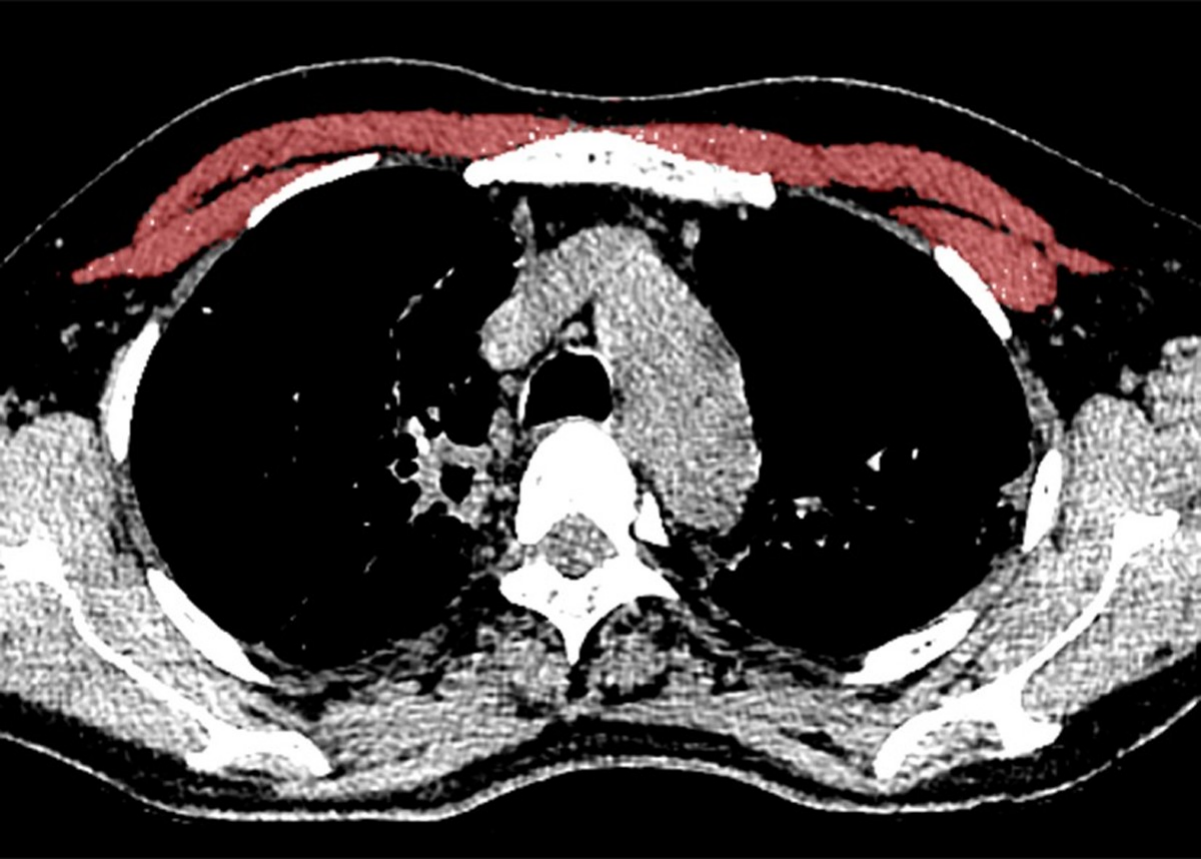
Measurement of pectoralis muscle area on chest CT. The pectoralis muscle area was quantitatively measured in the axial CT (3mm slice thickness) with standard reconstruction algorithm and mediastinal setting. The bilateral pectoralis muscle area (pectoralis major and minor) shaded in purple.
